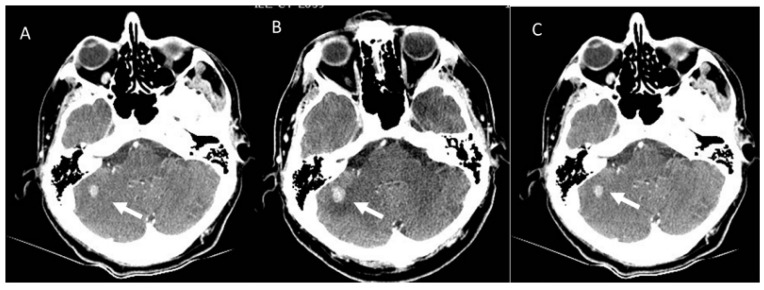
Pseudoprogression of brain metastasis (arrows) in an NSLC patient treated with immunotherapy. CT evaluation (A) in pre-treatment phase, after 1 month (B), and after 12 weeks of the first CT evaluation (C).
